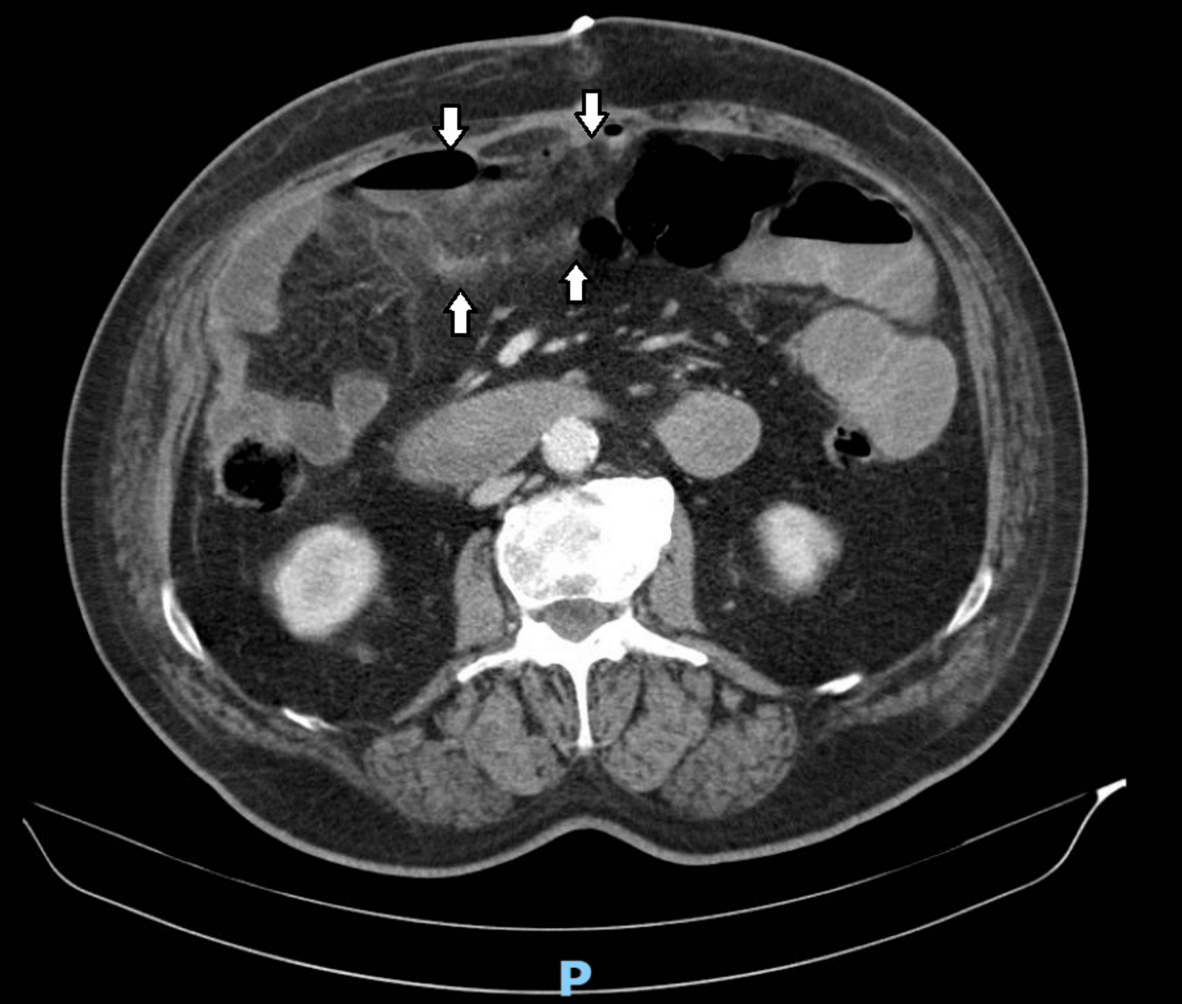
CT scan image revealing fat stranding and a small volume of loculated fluid suggesting a collection approximately 30 mm in diameter
